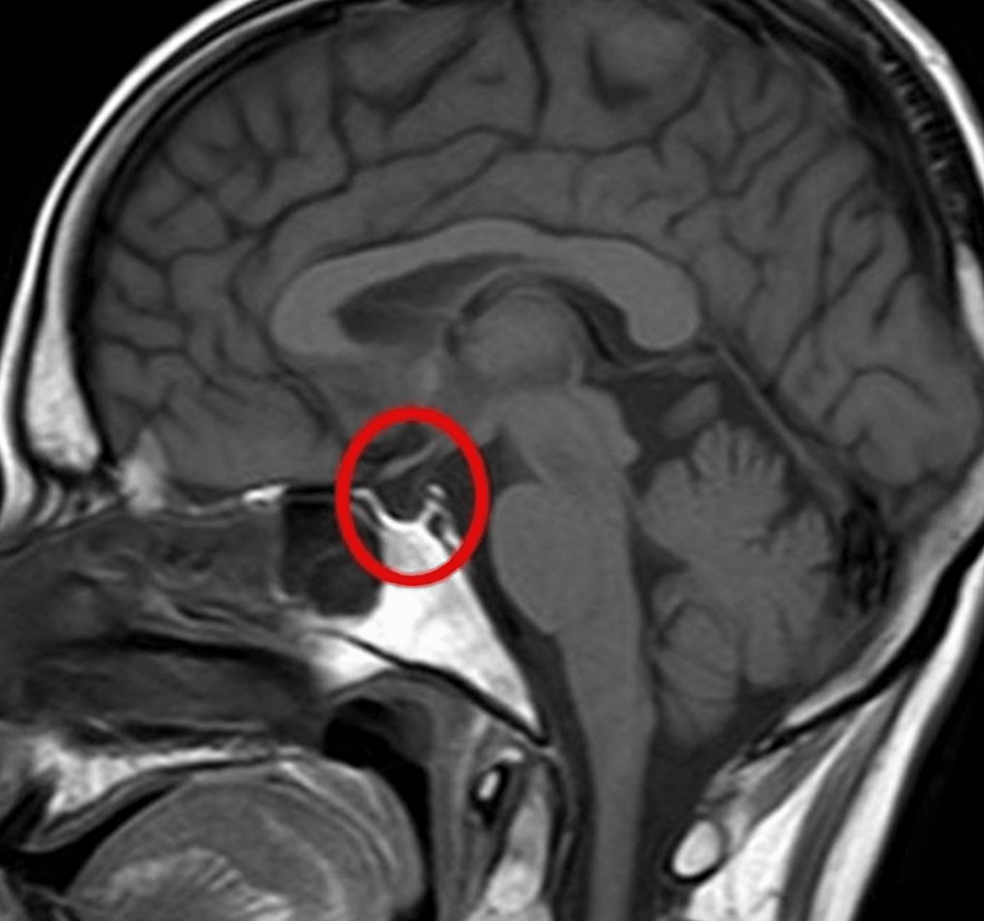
Brain MRI with the circle showing mild empty sella turcica.
